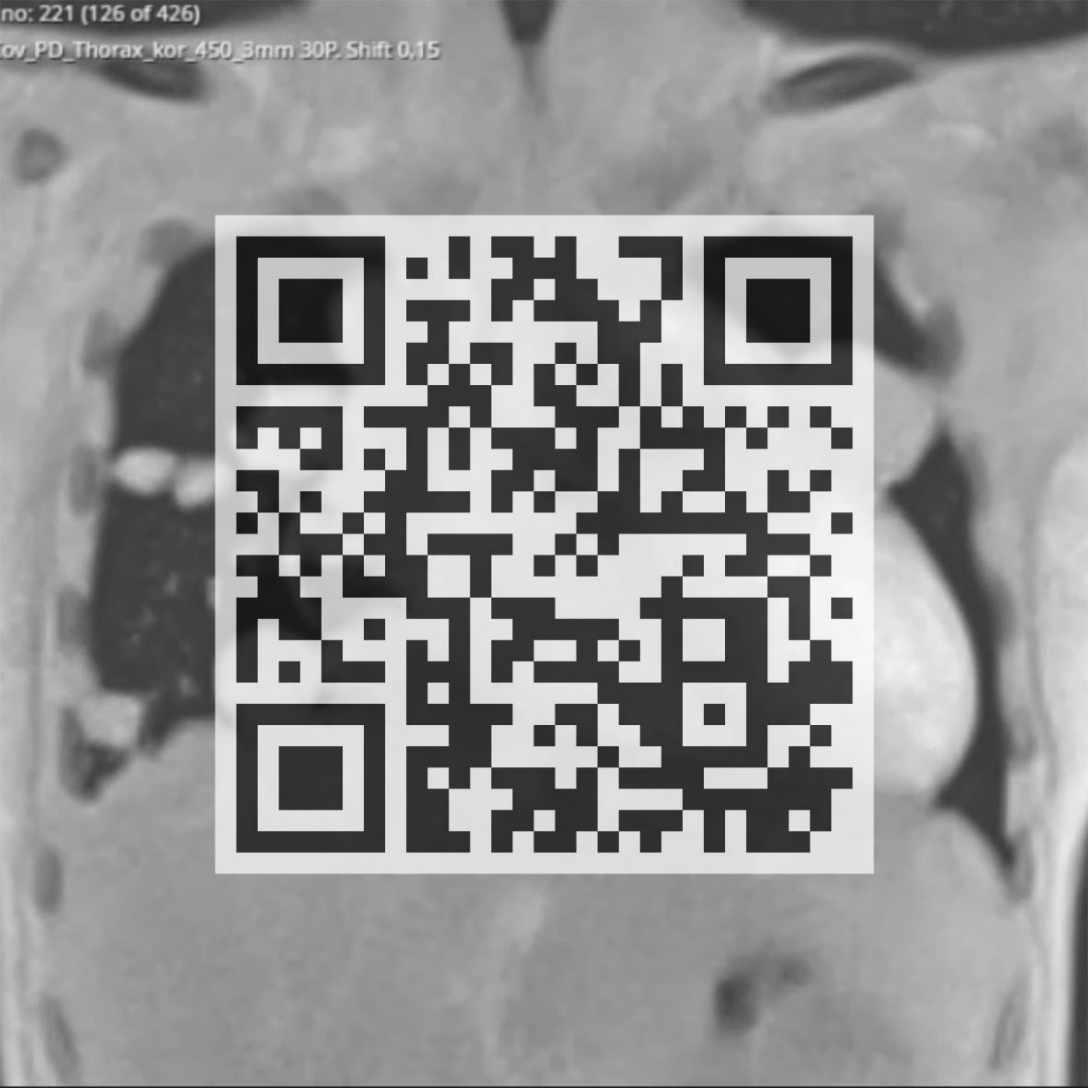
Video 7 Proton-density weighted volume coverage sequence in coronal orientation (video on the right side) in a 14-year-old girl with multiple lung metastases from sarcoma in the lower leg in comparison with the x-ray (on the left side)
